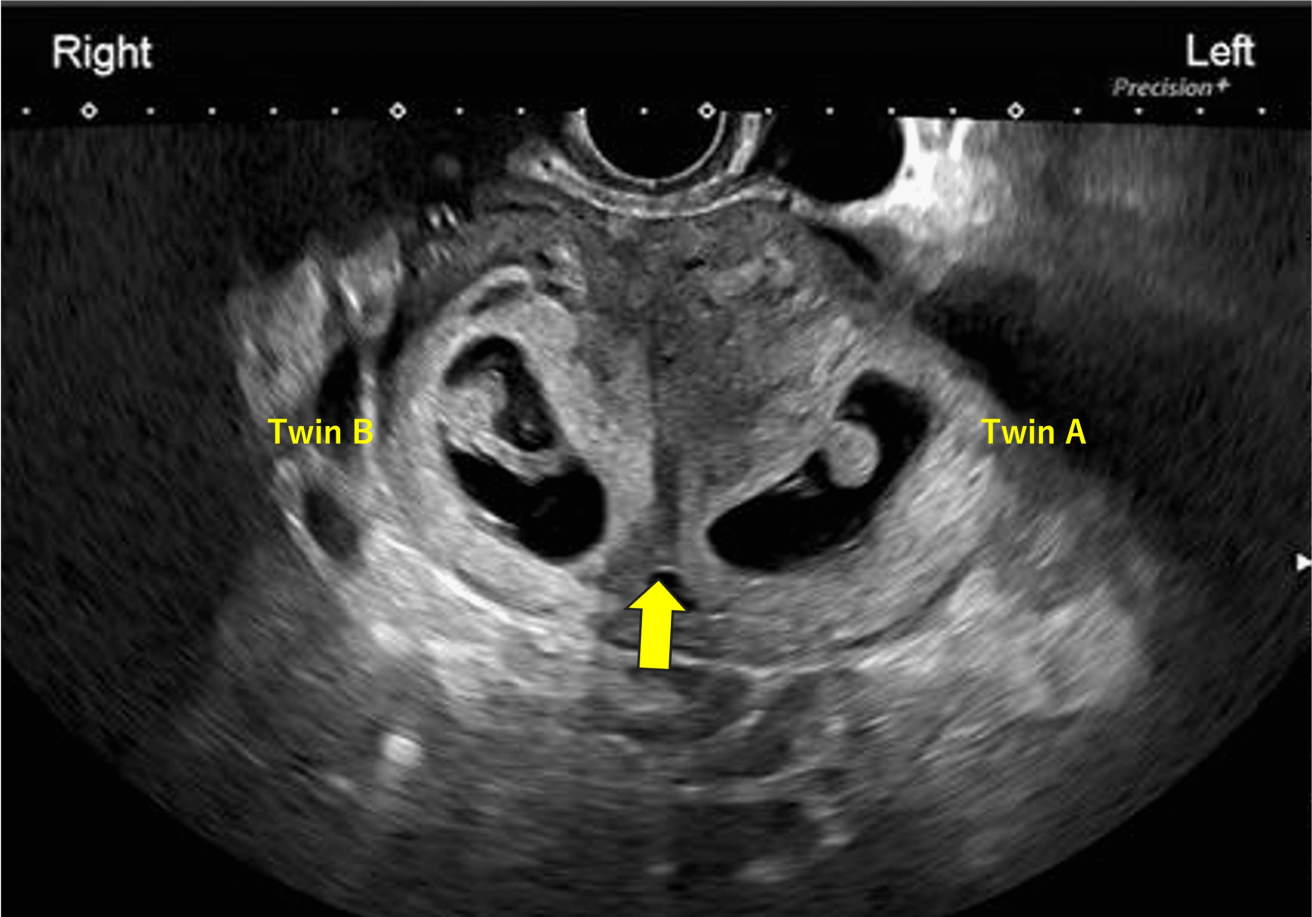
Transvaginal ultrasougraphy image at eight weeks of pregnancy. Gestational sac of Twin A was observed in the left uterine cavity, and Twin B  in the right, separated by the septum (yellow arrow).
